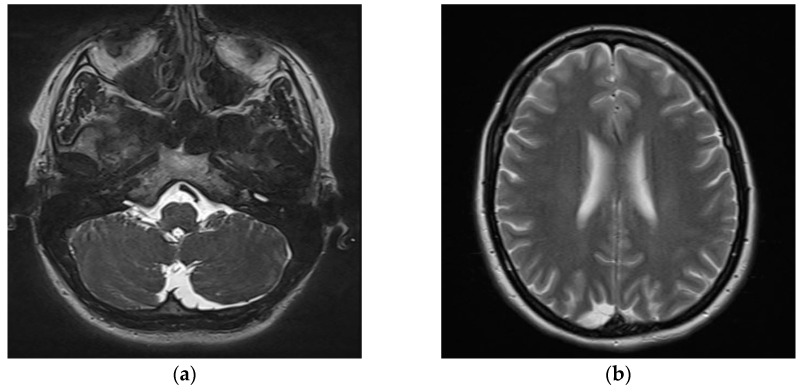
MRI scan showing: (a) Axial T2-weighted MRI of the posterior fossa and cerebellopontine angle; (b) Axial T2-weighted MRI of the cerebral hemispheres.
